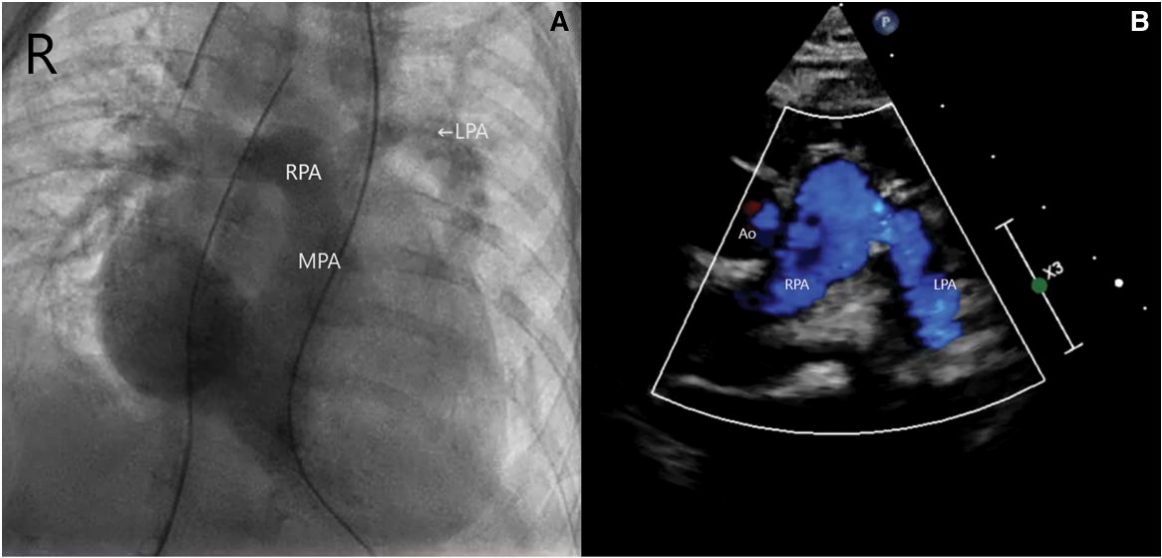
(*A* and *B*) Post-operative fluoroscopy demonstrates confluent branch pulmonary arteries. This was also demonstrated on echocardiography, with non-turbulent flow across both PAs seen. This is also demonstrated on fluoroscopy. RPA, right pulmonary artery; MPA, main pulmonary artery; LPA, left pulmonary artery; Ao, aorta.
